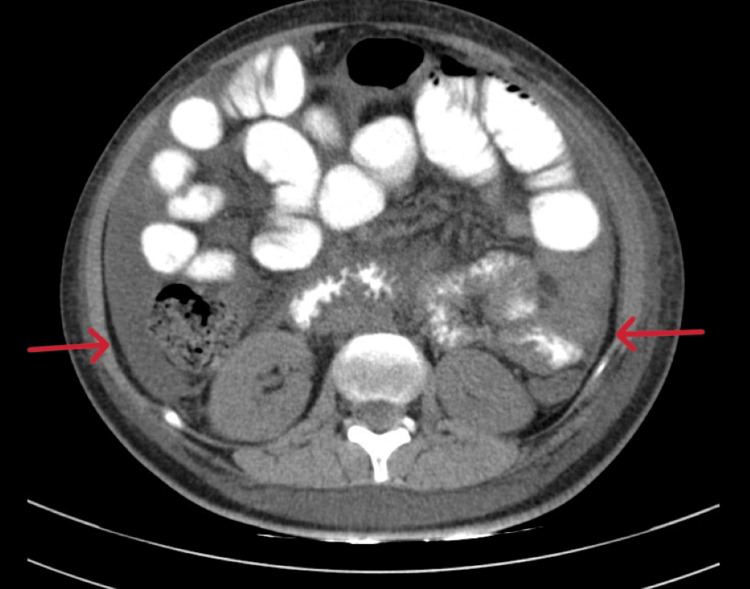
CECT of the abdomen and pelvis showing mild ascites (indicated by the red arrows). CECT: Contrast-enhanced computed tomography.
